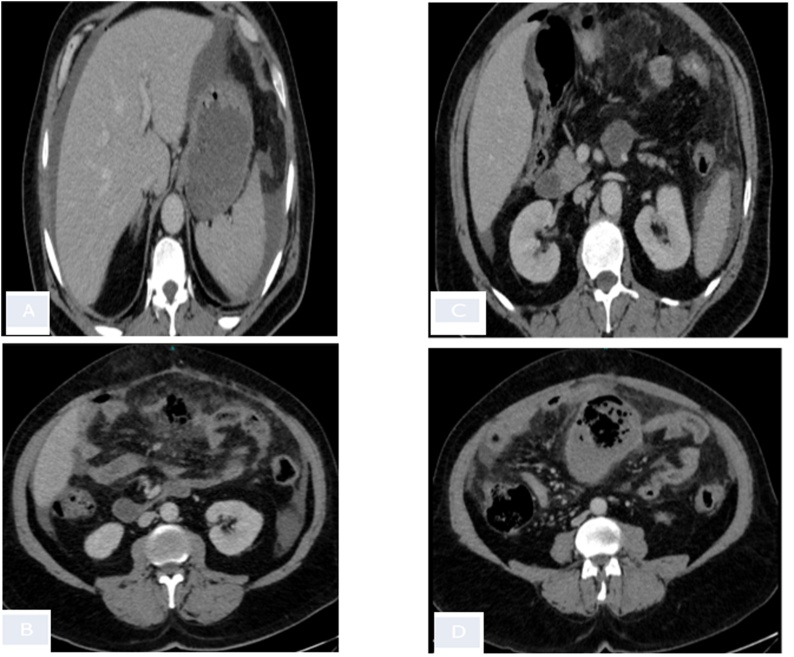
Abdominal CT scan. (A) Axial CT image demonstrated an intra-abdominal free fluid. (B) Axial CT image demonstrated a pneumoperitoneum and significant fat stranding in the small bowel mesentery. (C) Axial CT image demonstrated a pneumoperitoneum and significant fat stranding surrounding a jujenal segment of small bowel, which shoes gas locules at its wall representing the site of perforation. (D) Axial CT image demonstrated a focal hypo-attenuating wall thickening of small bowel segment and air fluid level.
